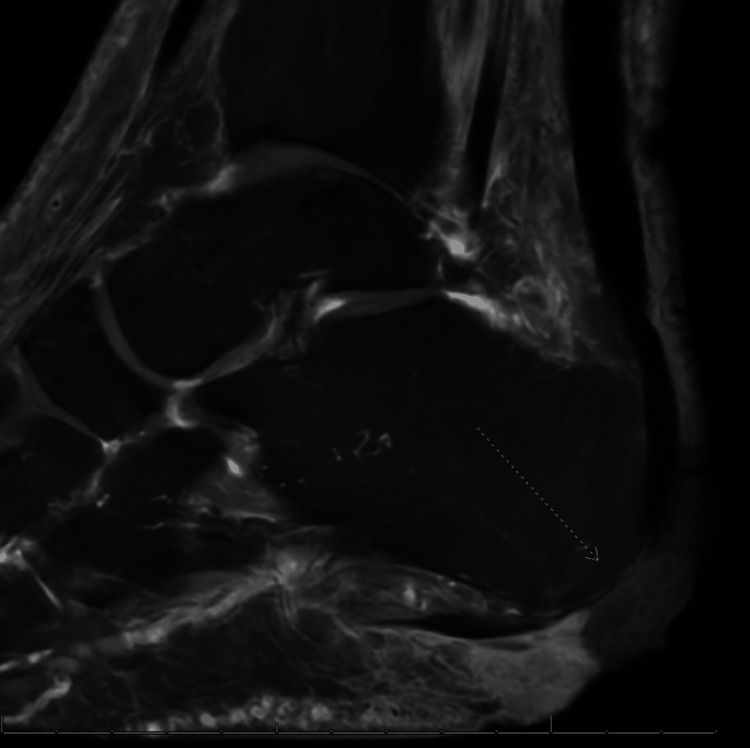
MRI of the calcaneus. This patient's MRI demonstrated osteomyelitis of the calcaneus (represented by the dotted arrow).
